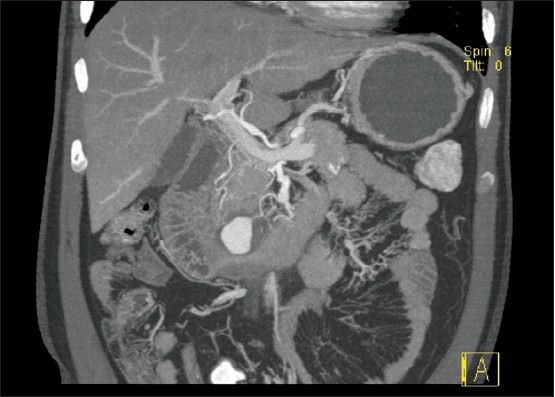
CT of the abdomen showing the PDA aneurysm.
